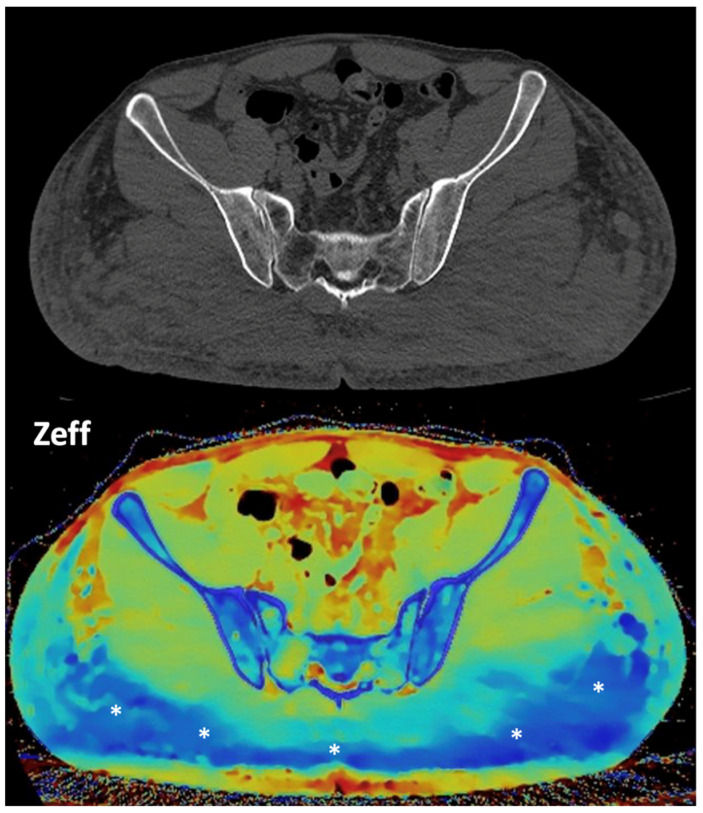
Gluteal silicone injection. Unenhanced CT image (top) shows a diffuse increase in density in both gluteal areas. Silicone deposition is better evaluated in a Zeff map (bottom), which separates silicone (blue areas, asterisks) from edema based on their different atomic numbers (silicon Z = 14).
